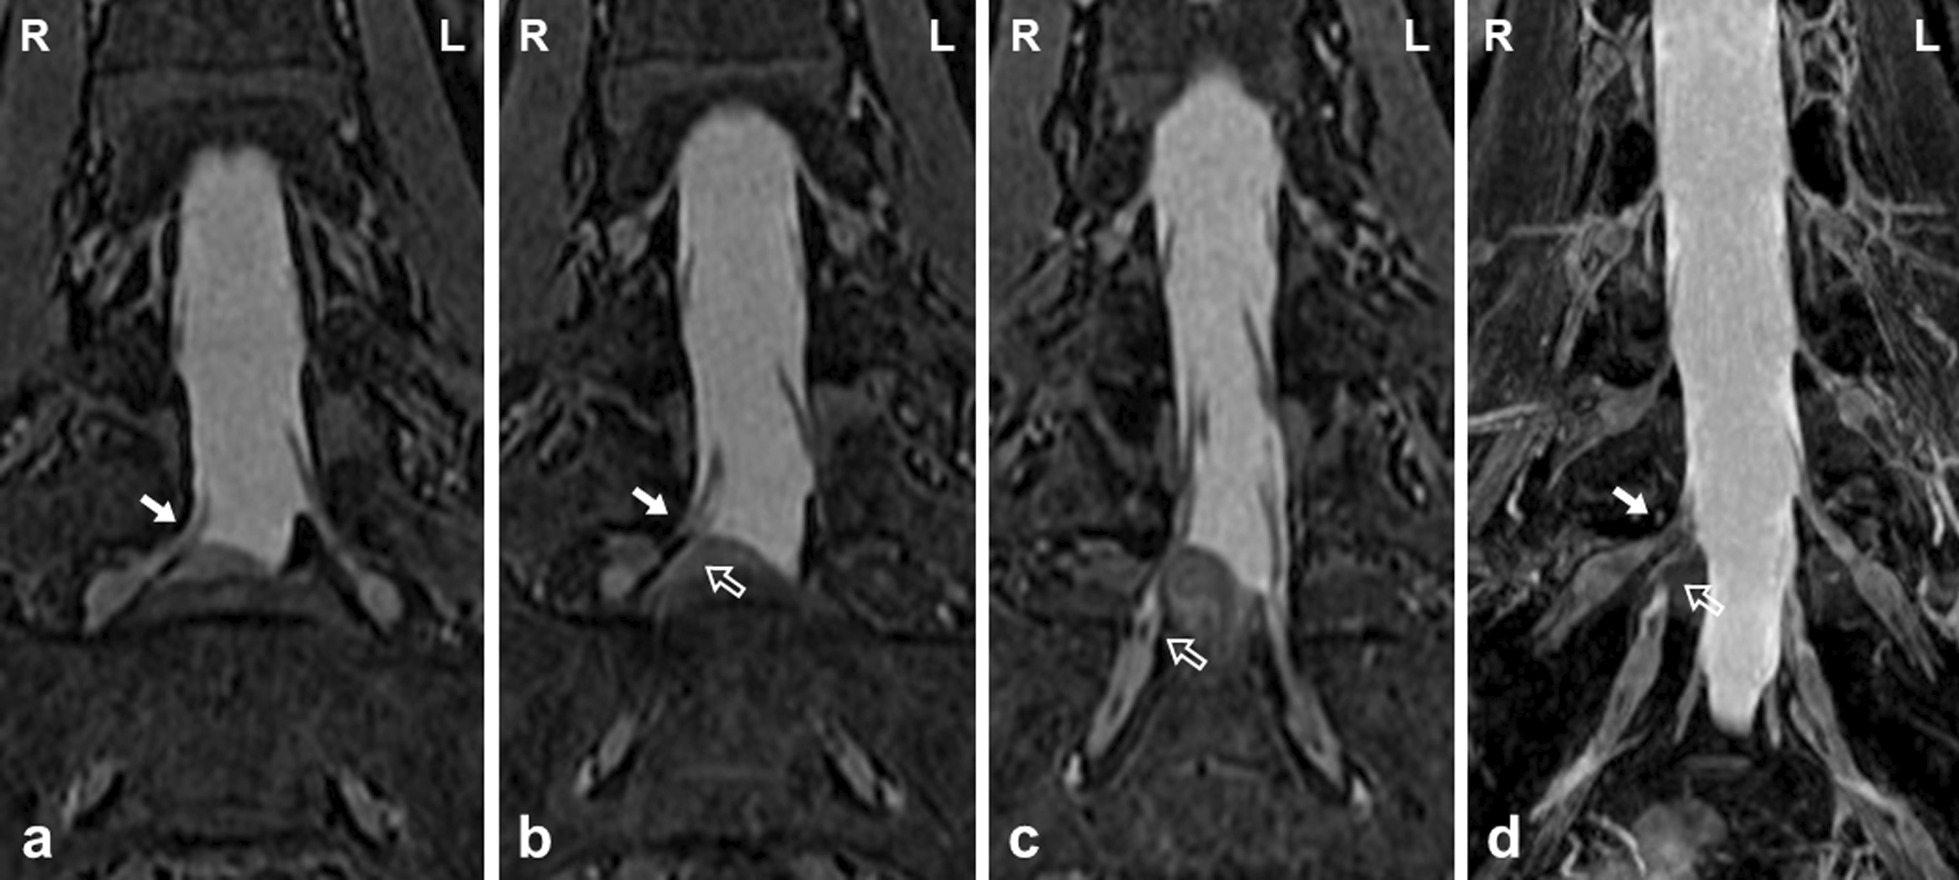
MRI. The laterality of the thickness and exit angle of the conjoined right L5–S1 nerve root was retrospectively confirmed (white arrow: right L5 root; open arrow: right S1 nerve root). a T2 coronal view at the L5 nerve root. b T2 coronal view at the L5/S1 nerve roots. c T2 coronal view at the S1 nerve root. d MR neurography
